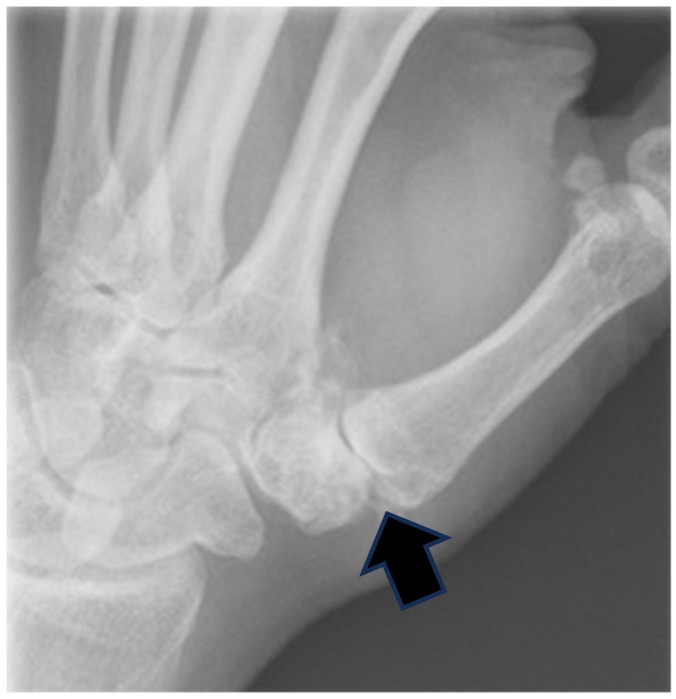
Preoperative radiograph of a 68-year-old female with Eaton stage III basal joint arthritis. The black arrow indicates the collapsed first carpometacarpal joint with osteophyte formation and subchondral sclerosis.
